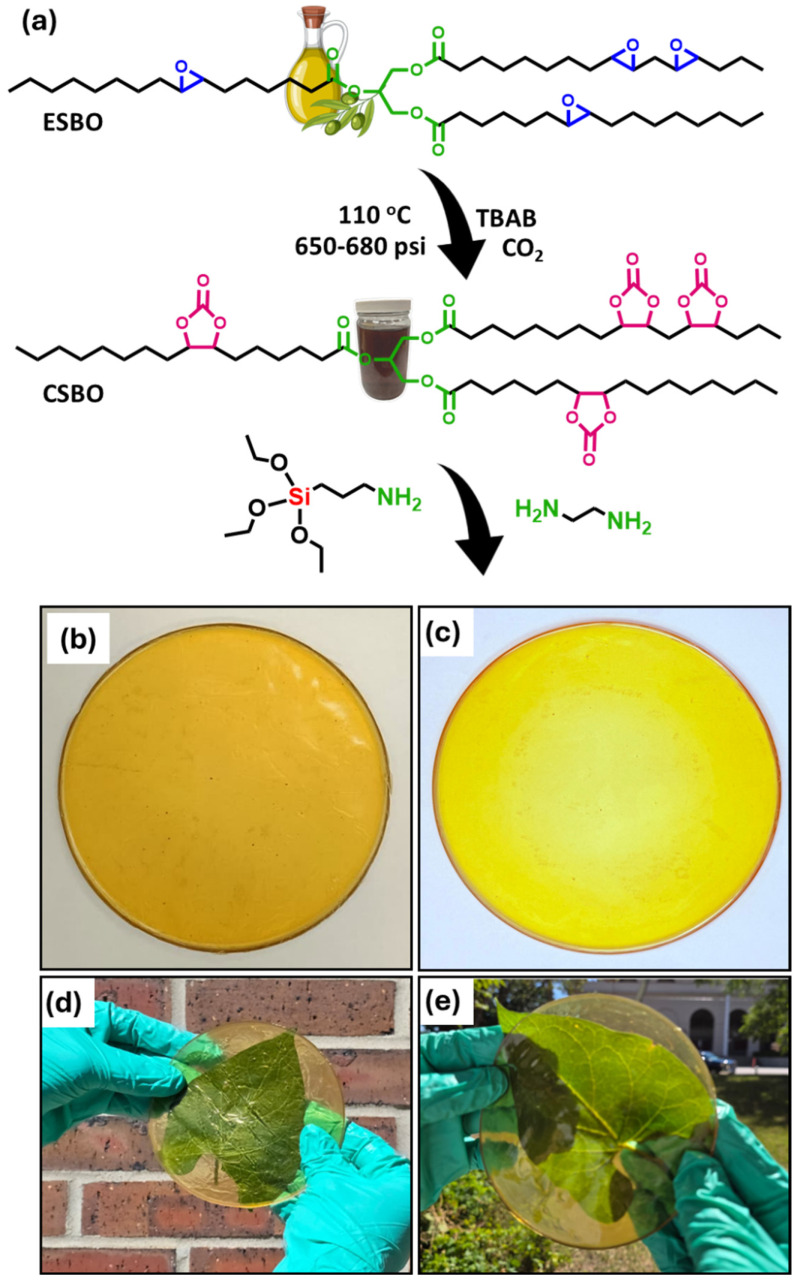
(a) Synthesis of CSBO and pictorial representation of the NIPU films. (b) CT. (c–e) CEA-5.
